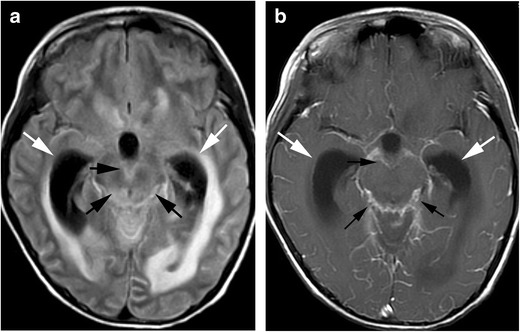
Pyogenic meningitis. An 8-year-old boy with acute myeloid leukaemia, who had received chemotherapy including intensification treatment, presented with esotropia. a FLAIR image shows hyperintensities along the surface of the brain stem (*arrows*). Communicating hydrocephalus is also seen (*white arrows*). b These lesions show enhancement (*arrows*)
